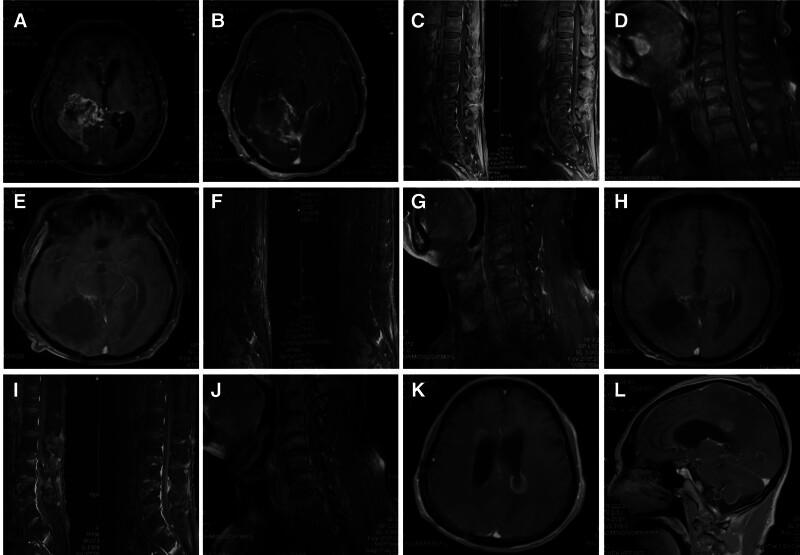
Imaging. (A) Preoperative head MRI shows an irregularly enhanced signal measuring 7.07 cm × 5.04 cm × 4.47 cm in the right hippocampus, temporal–occipital lobe, thalamus, corona radiata, and the splenium of the corpus callosum, invading the right lateral ventricle. (B) Postoperative 1-month head MRI demonstrates complete resection of the tumor. (C) Postoperative 2-month lumbar MRI reveals small nodular abnormal enhancement at the L2–3, L4–5 intervertebral disc levels and S2 vertebral body level in the spinal canal. (D) Cervicothoracic MRI shows a significant enhancing nodular signal in the spinal canal at the T2 vertebral body level. (E) Postoperative 5-month head MRI shows a reduction in both the original abnormal signal and surrounding enhanced signal. (F) Postoperative 5-month lumbar MRI shows no significant change in the abnormal enhancement signal within the lumbar spinal canal. (G) Postoperative 5-month cervicothoracic MRI shows a reduction in the abnormal enhancement signal in the spinal canal at the T2 vertebral body level. (H) Postoperative 8-month head MRI shows further reduction in the intensity and extent of the original abnormal signal. (I, J) Postoperative 8-month cervicothoracic MRI reveals no significant enlargement of the original abnormal signal in the spinal canal. (K, L) Postoperative 10-month head MRI shows abnormal enhancement signals in multiple areas of the cerebral cortex and periventricular regions consistent with tumor presence.
